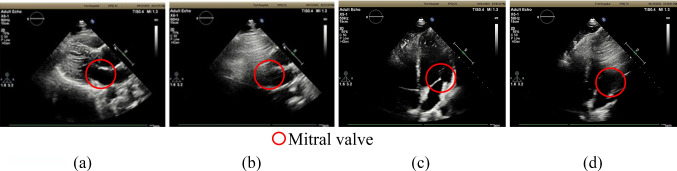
Ultrasound images in sitting posture; a clear parasternal long-axis view; b unclear parasternal long-axis view; c clear apical four-chamber view; d unclear apical four-chamber view
